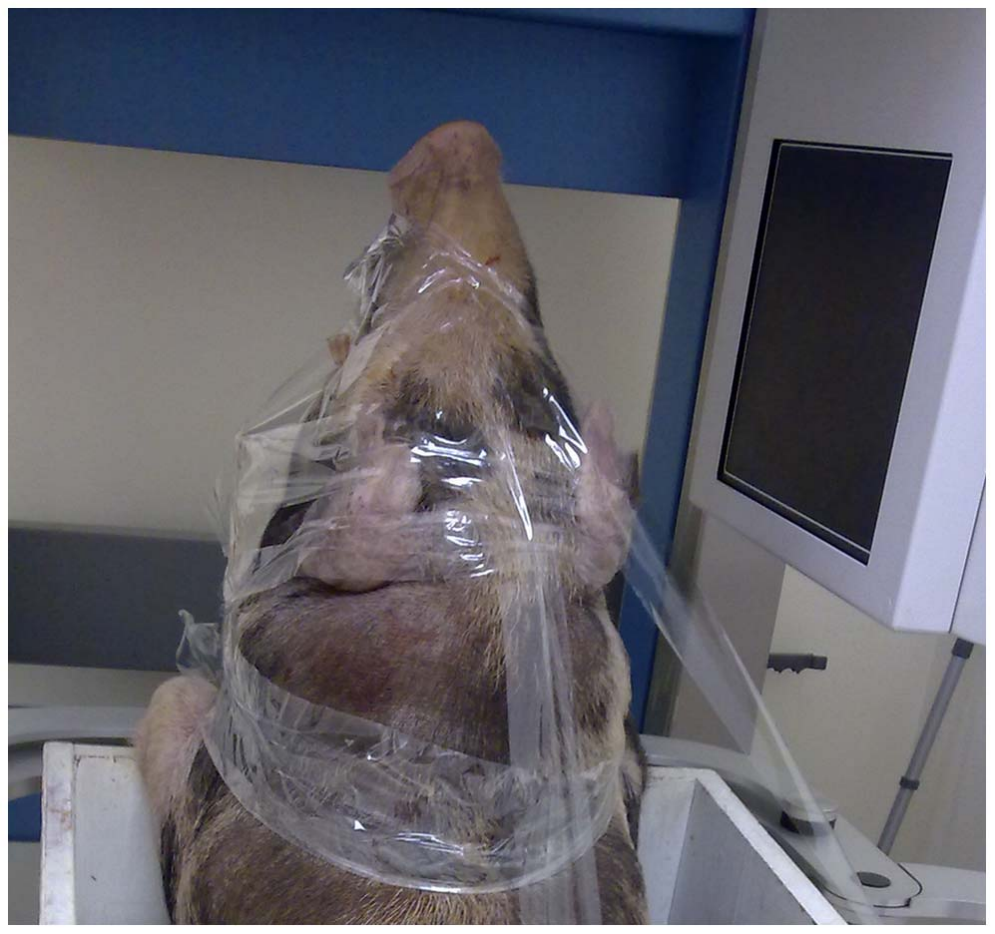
The experimental setup for the CBCT scan of a typical subject.
